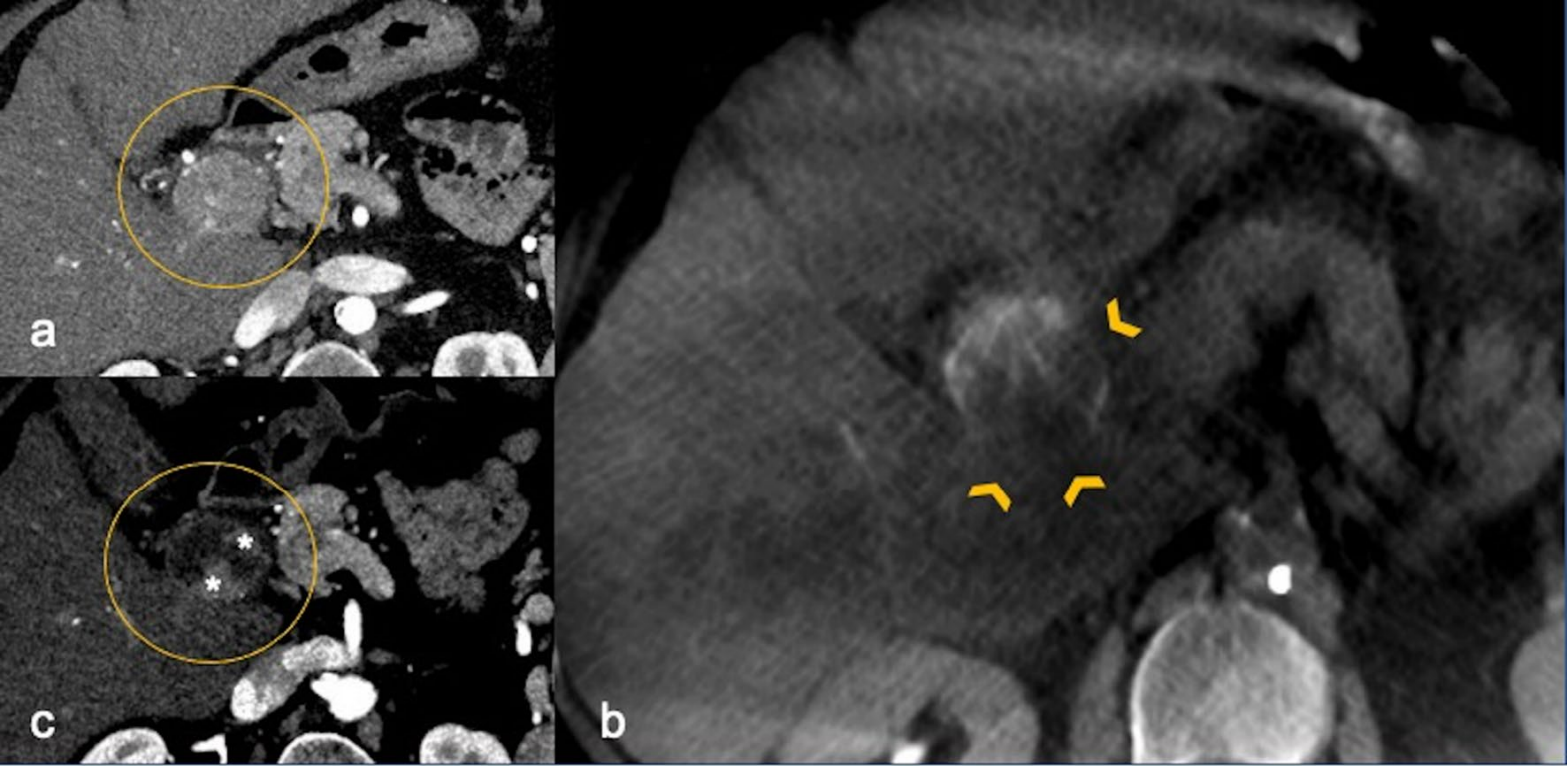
70-year-old male. a Pre-procedural axial arterial phase of contrast-enhanced CT scan showing a 4.3 cm exophytic HCC (circle) in IV hepatic segment. The patient underwent combined treatment: (b) the axial unenhanced CBCT image shows a partial Hug Sign–beads/trapped contrast agent incompletely surrounding the post-ablation volume (180°) (arrowheads). c 2-months follow-up axial arterial phase contrast-enhanced CT scan showing eccentric residual tumor (*) consistent with partial response. HCC: hepatocellular carcinoma. CBCT: cone-beam CT
